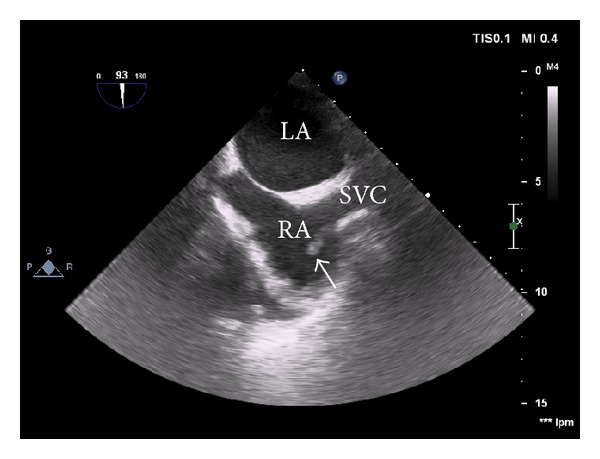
Transesophageal echocardiography showing a tubular 4 × 0.5 cm thrombus (arrow) in superior vena cava (SVC) to the right atrium (RA); left atrium (LA).
